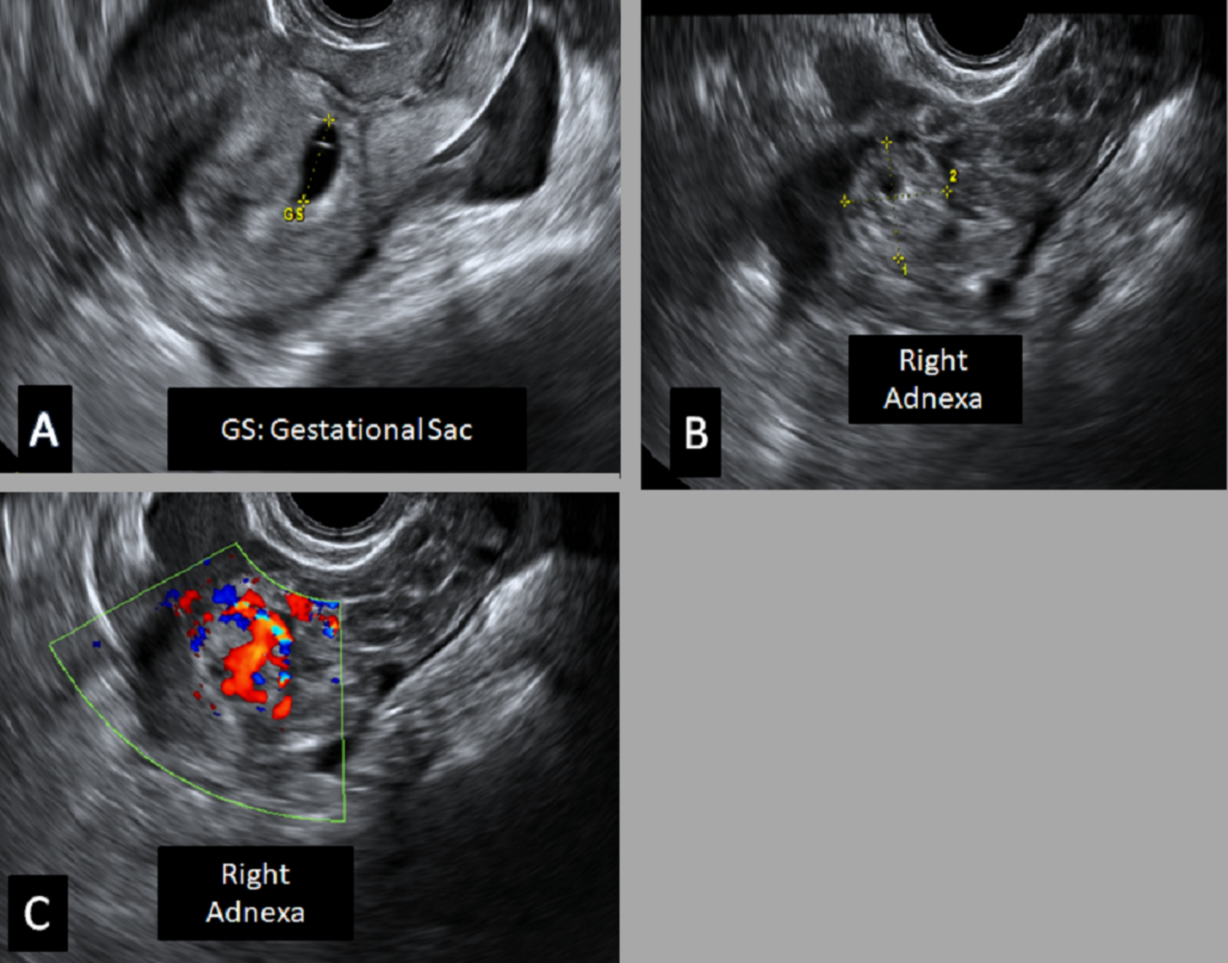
Transvaginal ultrasound A) Intrauterine elongated gestational sac (GS); B-C) There is a right adnexal complex lesion containing rounded thick-walled echogenic structure; Doppler study revealed a ring of fire appearance, likely a picture of right adnexal ectopic pregnancy with hematoma favoring the diagnosis of heterotopic pregnancy.
